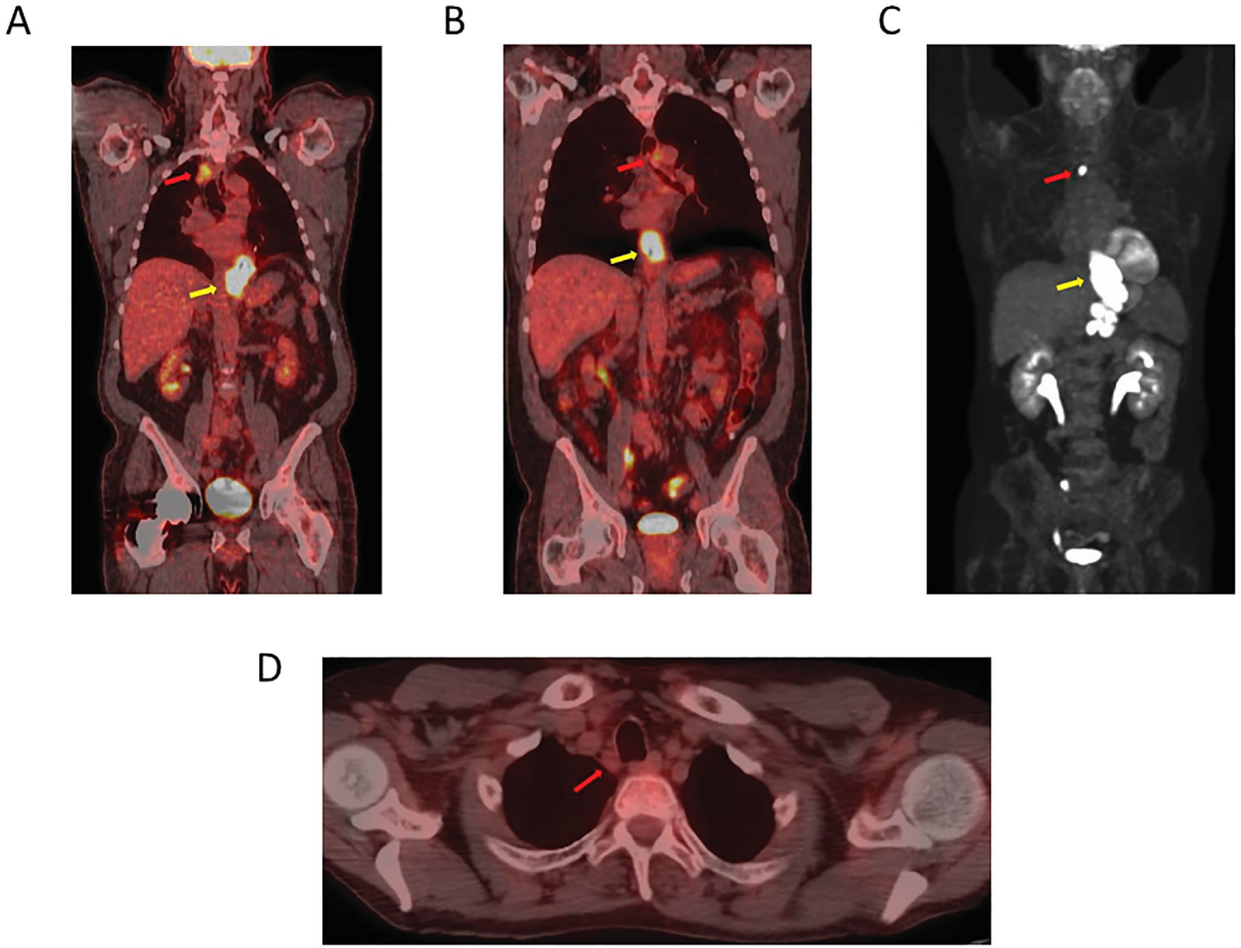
A PET-CT image showing a lower esophageal/GEJ primary cancer A. An avid high right upper mediastinal node. B. An avid high left upper mediastinal node. C. An avid high mediastinal node (towards right side). D. A right paratracheal non-avid node.
